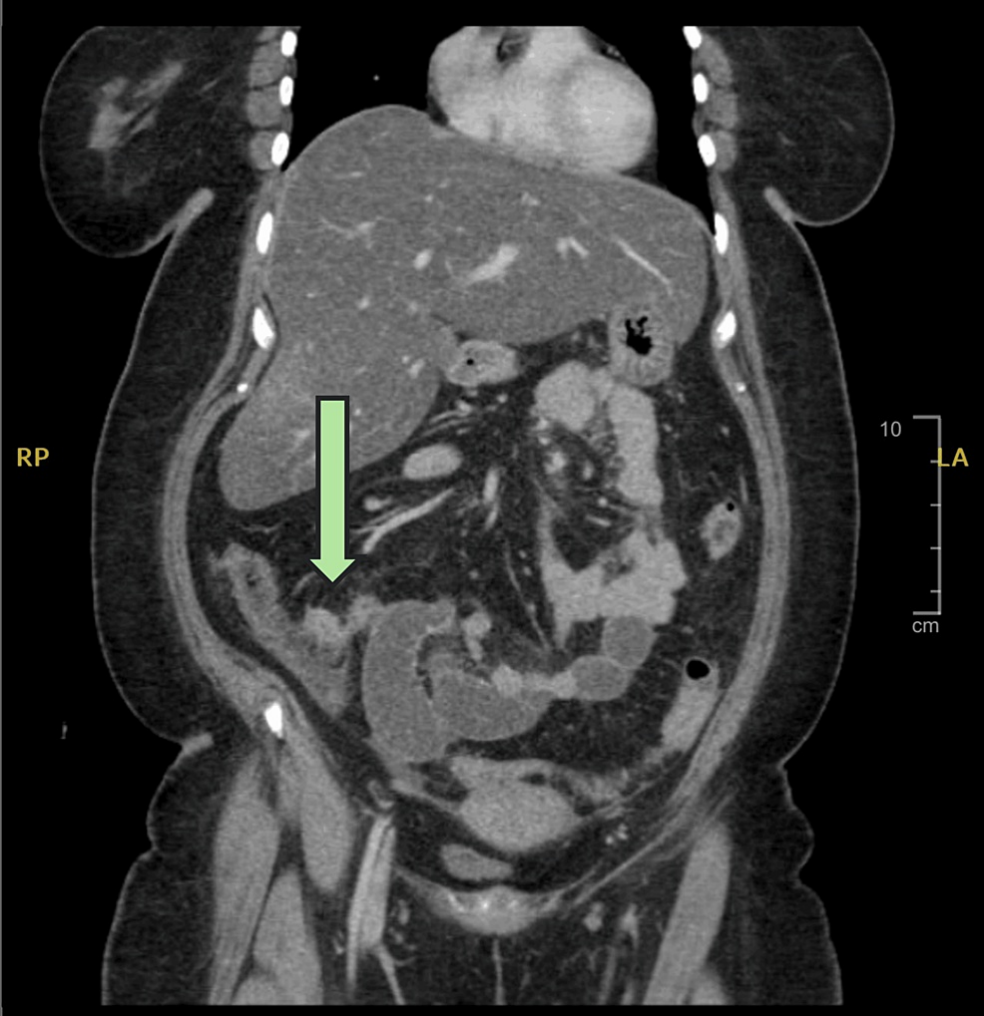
CT abdomen with contrast CT: Computed tomography The arrow indicates the enhanced structure with wall discontinuity.
